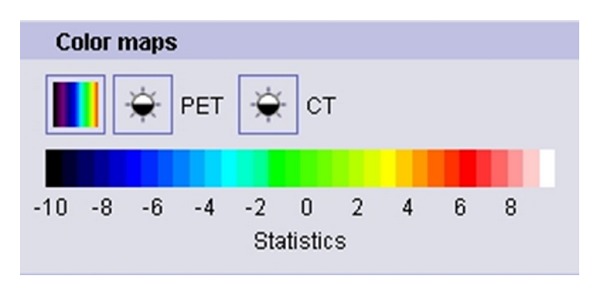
French Color coding for statistical reconstruction of the PET-CT scan images.
